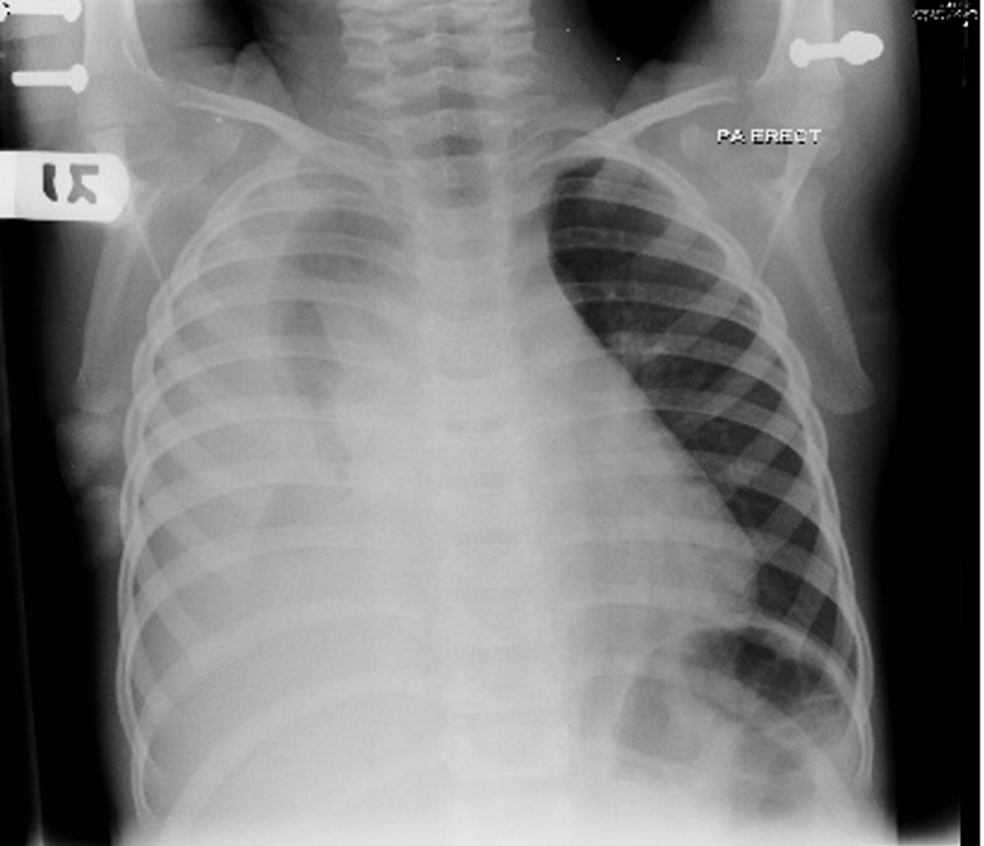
Plain chest X-ray, posteroanterior view, showing a diffuse opacity occupying most of the right-sided chest obscuring the costophrenic and the cardio-phrenic angle, a tracheal deviation to the left side with absence of air bronchogram, and positive meniscus sign.
